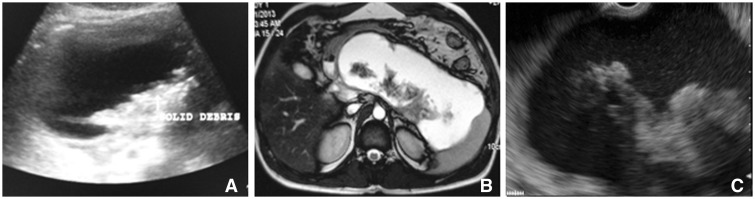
Imaging in patient with WOPN having 10–40% solid necrotic debris. a) Abdominal ultrasound; b) Magnetic resonance imaging; c) Endoscopic ultrasound.
